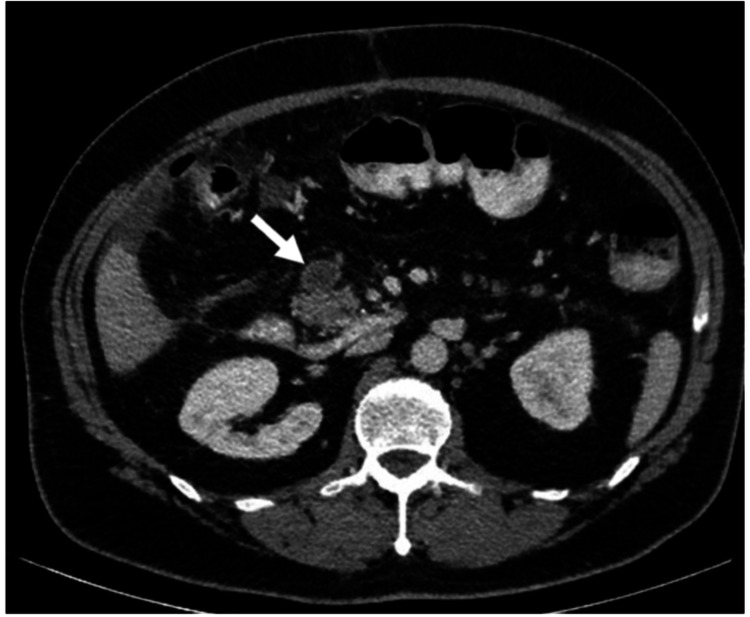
Abdominal CT scan in the portal phase demonstrating complete thrombosis of the superior mesenteric vein (arrow).
